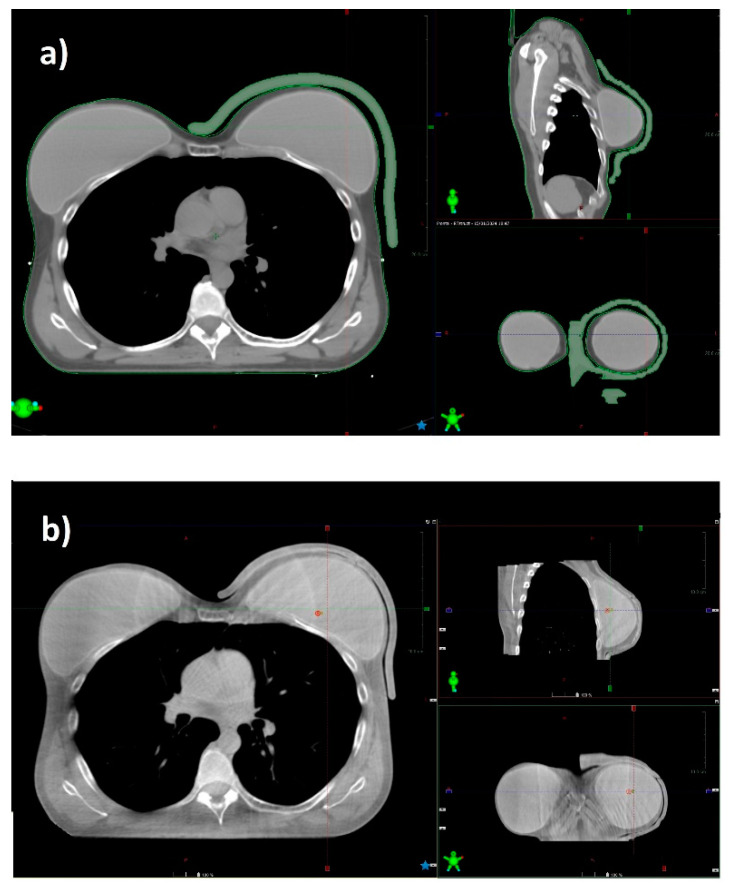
CT scan of a patient with a prosthesis and a standard gel bolus (a), and the CBCT acquired before radiation delivery in the same patient (b). The 3D bolus fits the skin without relevant air gaps.
